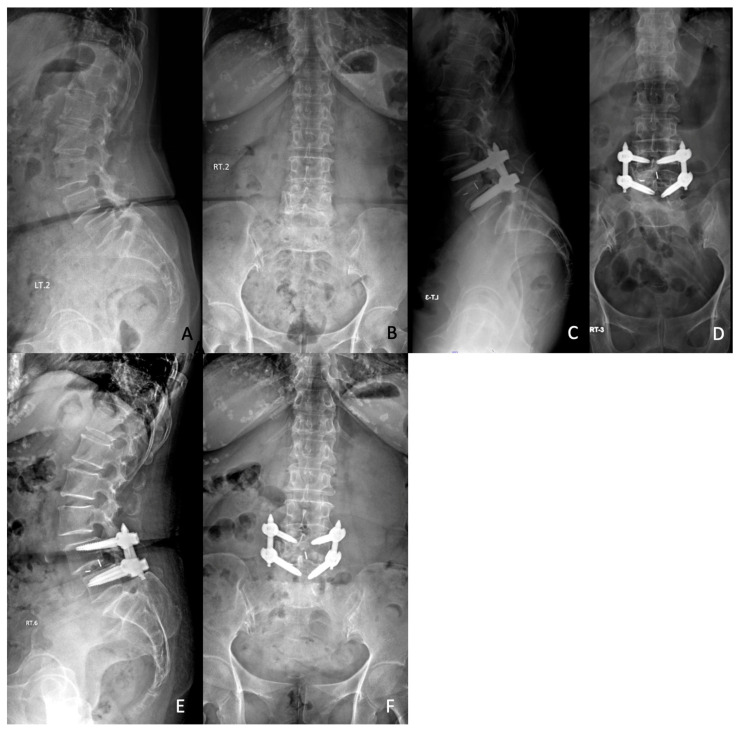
Representative case of fixation failure in a 71-year-old female with high pelvic incidence. (A,B) Preoperative standing radiographs demonstrate a high PI (71°) with degenerative spondylolisthesis. (C,D) Immediate postoperative images show satisfactory reduction and restoration of lumbar lordosis, with improved sagittal alignment. (E,F) At 6 months postoperatively, radiographs reveal screw loosening with loss of reduction and recurrent listhesis (7.53 mm).
